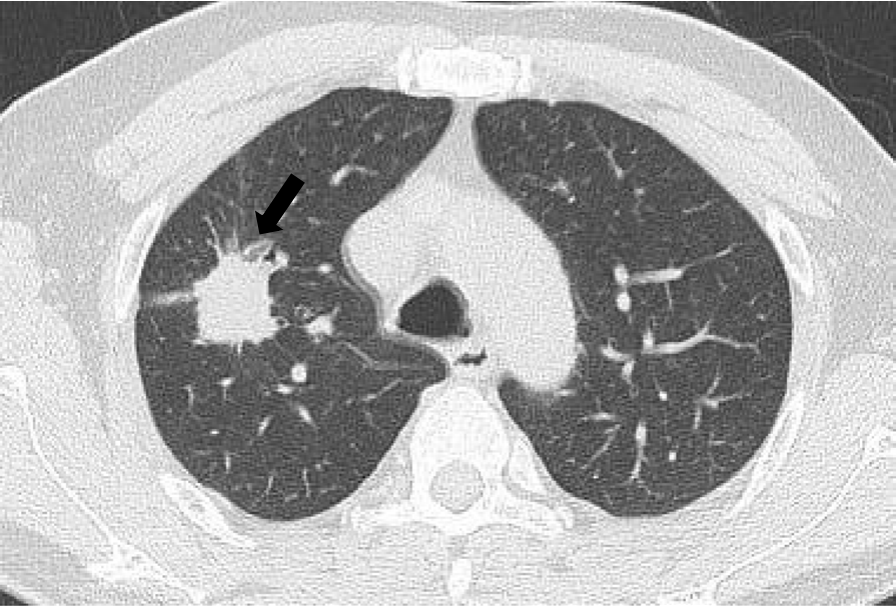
Solid nodule with ALK rearrangement. Axial CT image in a patient with ALK-positive adenocarcinoma showed a typical solid right upper lobe mass (arrow) with lobulation and spiculation
